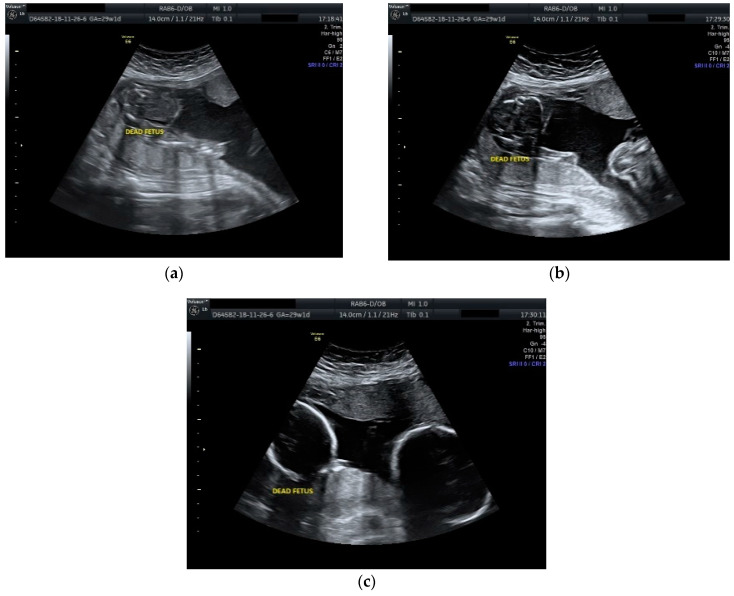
Ultrasound scan performed at 29 weeks and 1 day of gestation: (a), (b) dead fetus along with normal growing fetus with normal amniotic fluid; (c) cephalic extremities of the two fetuses with evident growth discordance.
